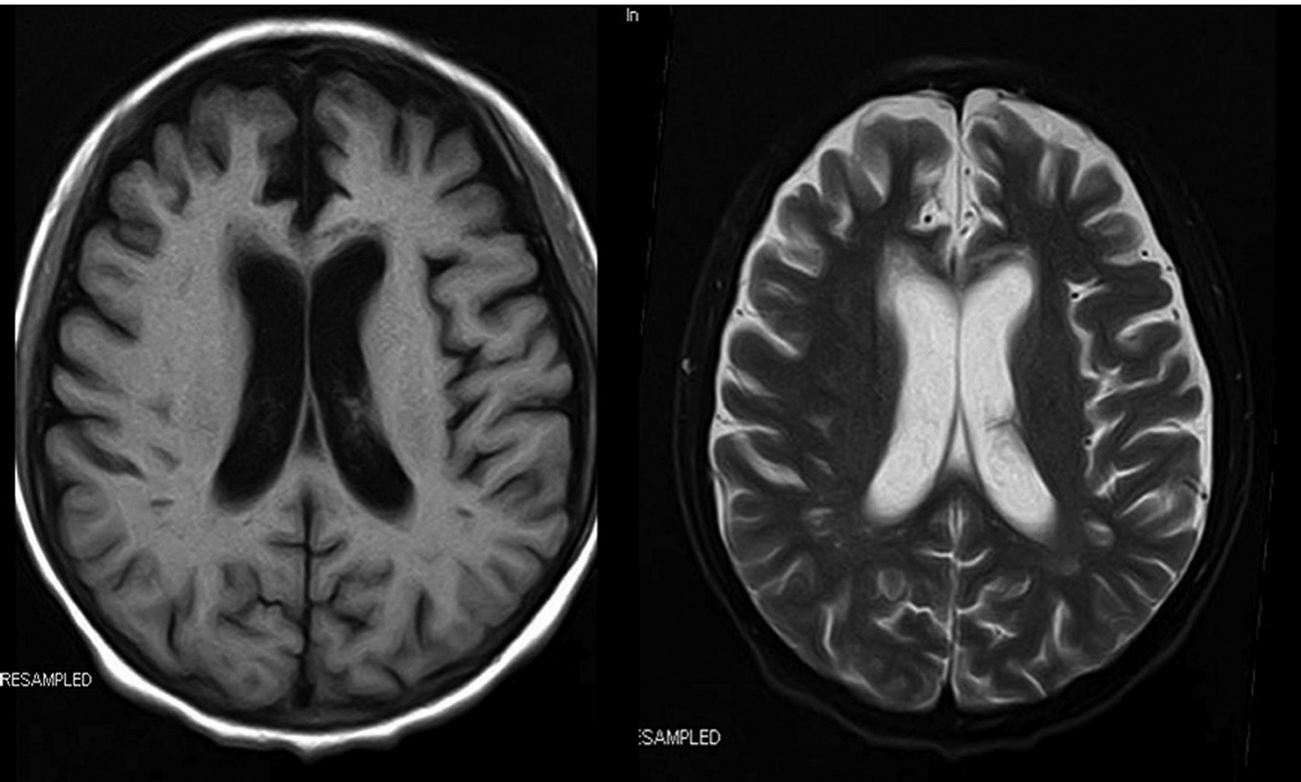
Brain MRI indicated cerebral atrophy after liver transplantation.
